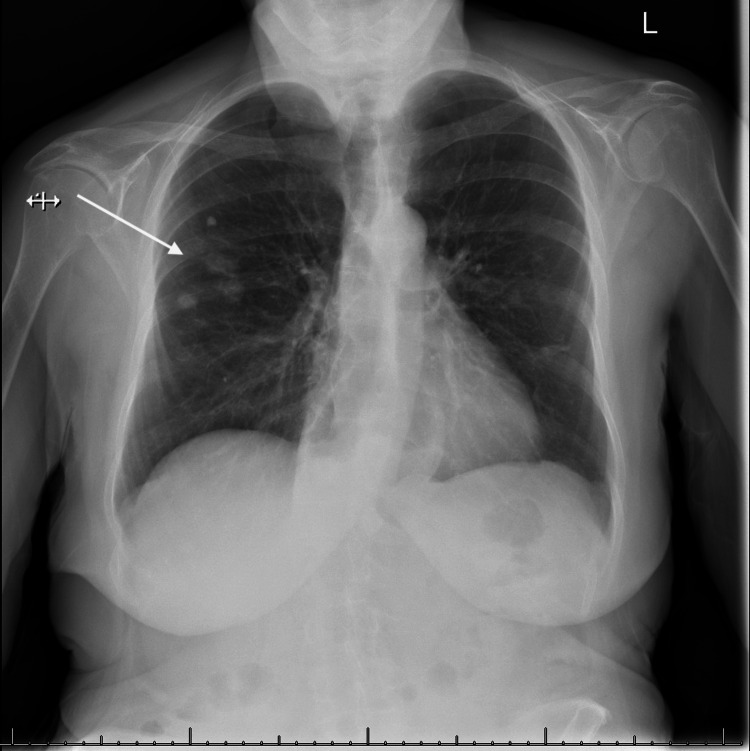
Chest x-ray showing nodular infiltrates in right lung fields
